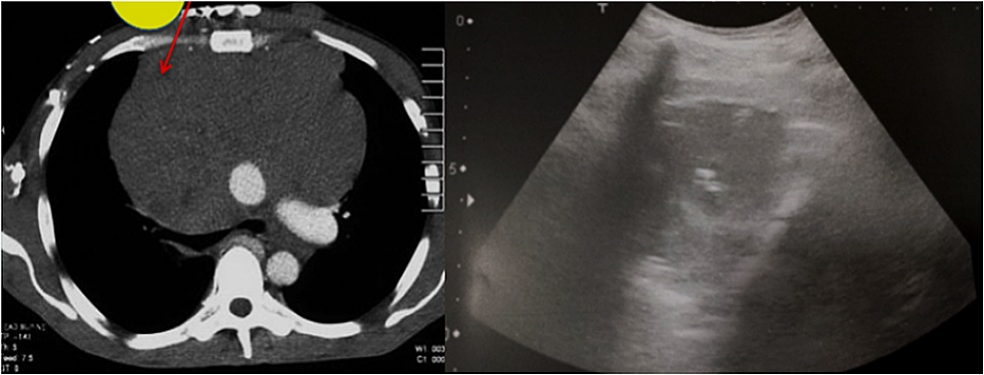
Plain CT scan of the chest showing a large anterior mediastinal mass. Ultrasound-guided para-sternal needle placement was performed for transthoracic mass biopsy.
